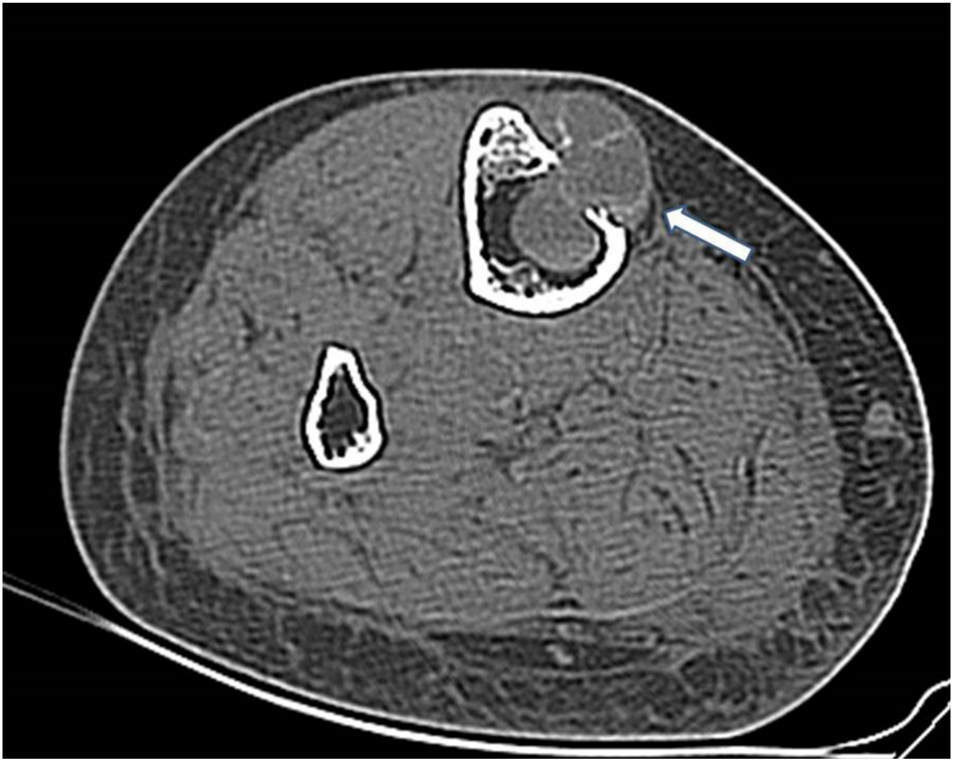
Case 3: CT scan of distal tibia: the similar lesion in distal end of the tibia
